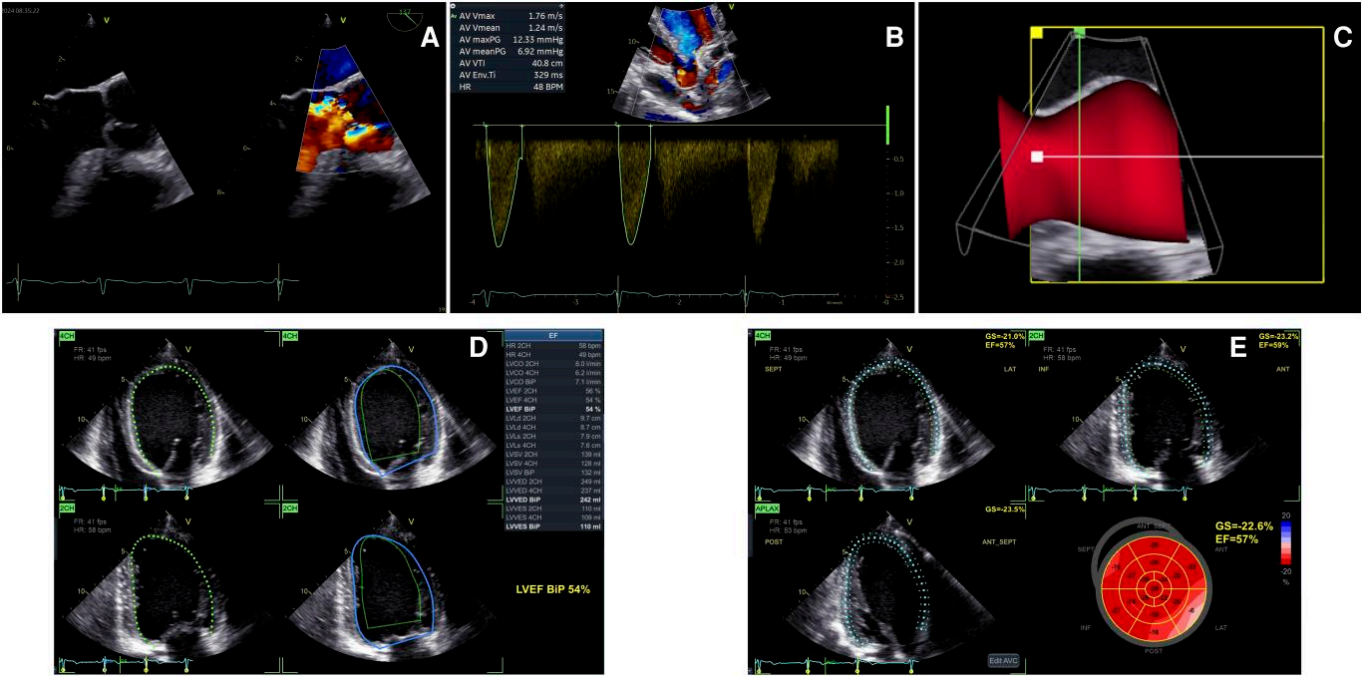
Artificial intelligence-powered echocardiographic assessment of a patient with bicuspid aortic valve and severe aortic regurgitation due to prolapse. (*A*) Prolapse of the anterior portion of the fused cusp causing severe eccentric aortic regurgitation. (*B*) Cardiac Auto Doppler function (GE HealthCare) to sample and average transaortic gradients to exclude aortic stenosis. (*C*) Semi-automated software (aortic valve quantification, GE HealthCare) three-dimensional reconstruction of the aortic root complex. (*D*) Automatic left ventricular volumes and ejection fraction estimation (Auto-EF, GE HealthCare) revealing a severe dilation and an ejection fraction at the lower normal limit. (*E*) Automatic global longitudinal strain calculation (Easy-AFI) demonstrating normal longitudinal contractility. AI, artificial intelligence; AR, aortic regurgitation; AVG, aortic valve quantification; 3D, three-dimensional; EF, ejection fraction; GLS, global longitudinal strain.
